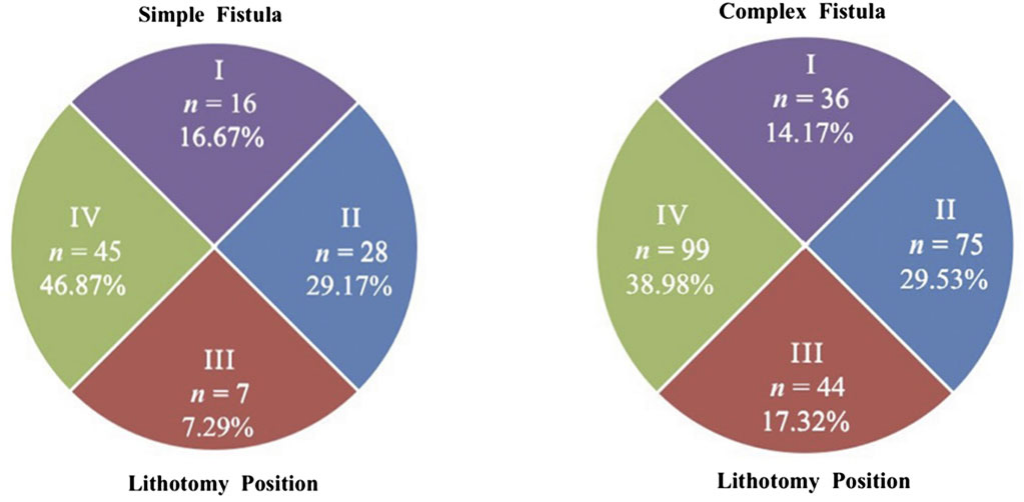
The distribution of internal opening (IO) locations in quadrants via transcutaneous perianal ultrasound. *n* refers to the number of IOs.
